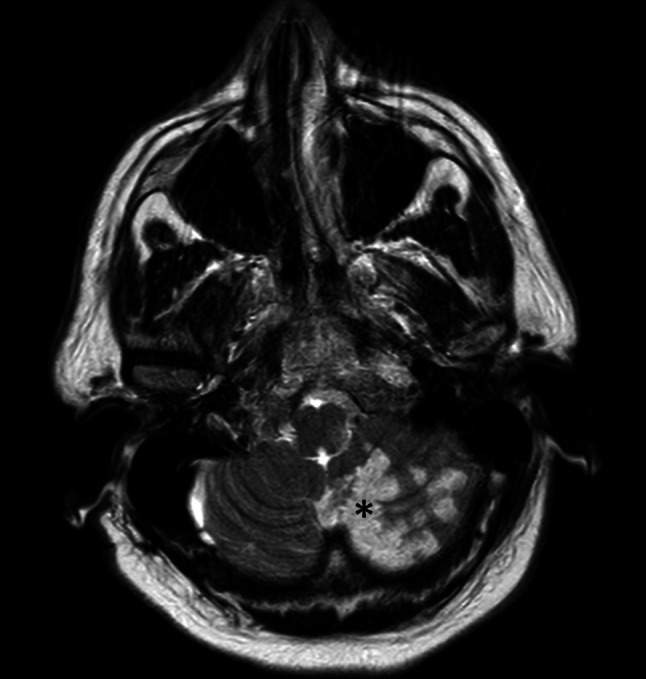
T2-weighted magnetic resonance imaging showing infarcted area (*asterisk*)
